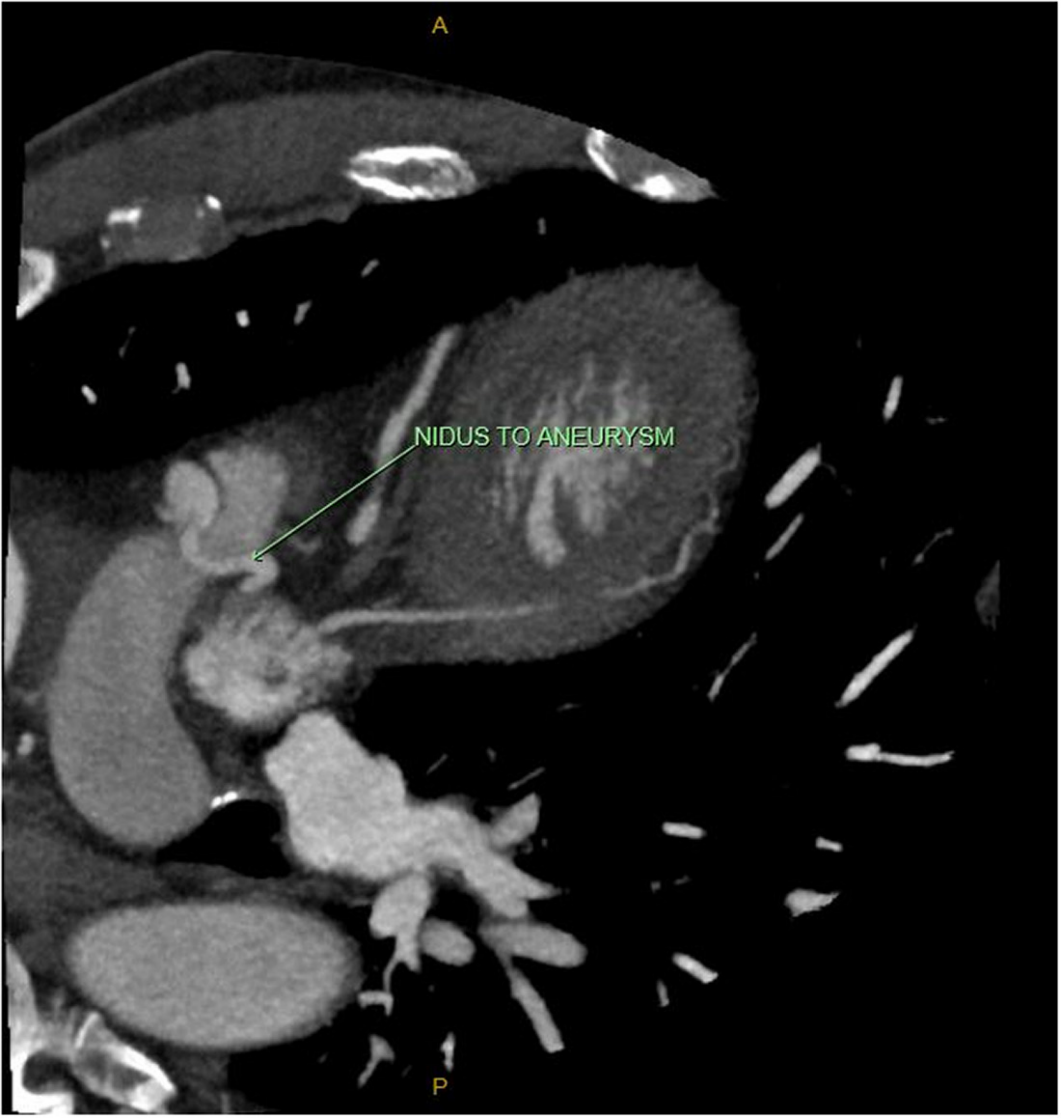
CTCA depicting vascular nidus of the fistula dilating into a focal 12 × 11 mm aneurysm in its distal segment. CTCA, CT coronary angiogram.
